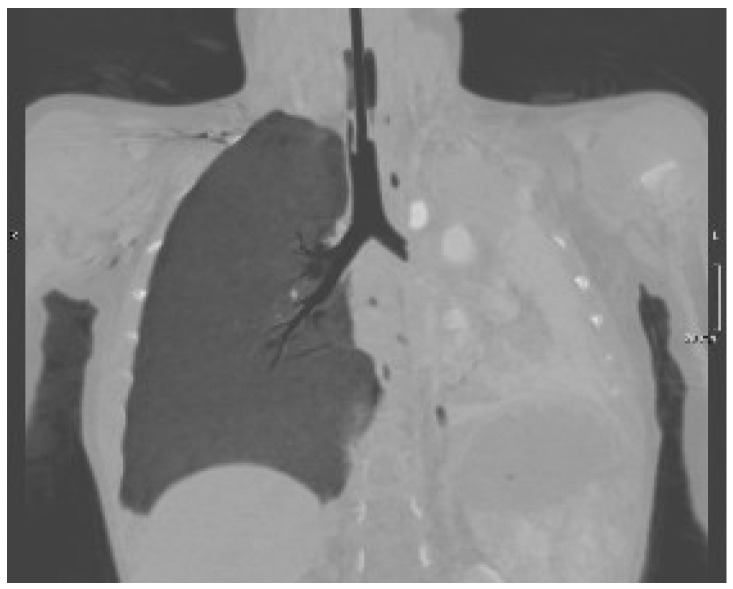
Chest CT scan showing a complete atelectasis of the left lung and an interruption of the main left bronchus.
